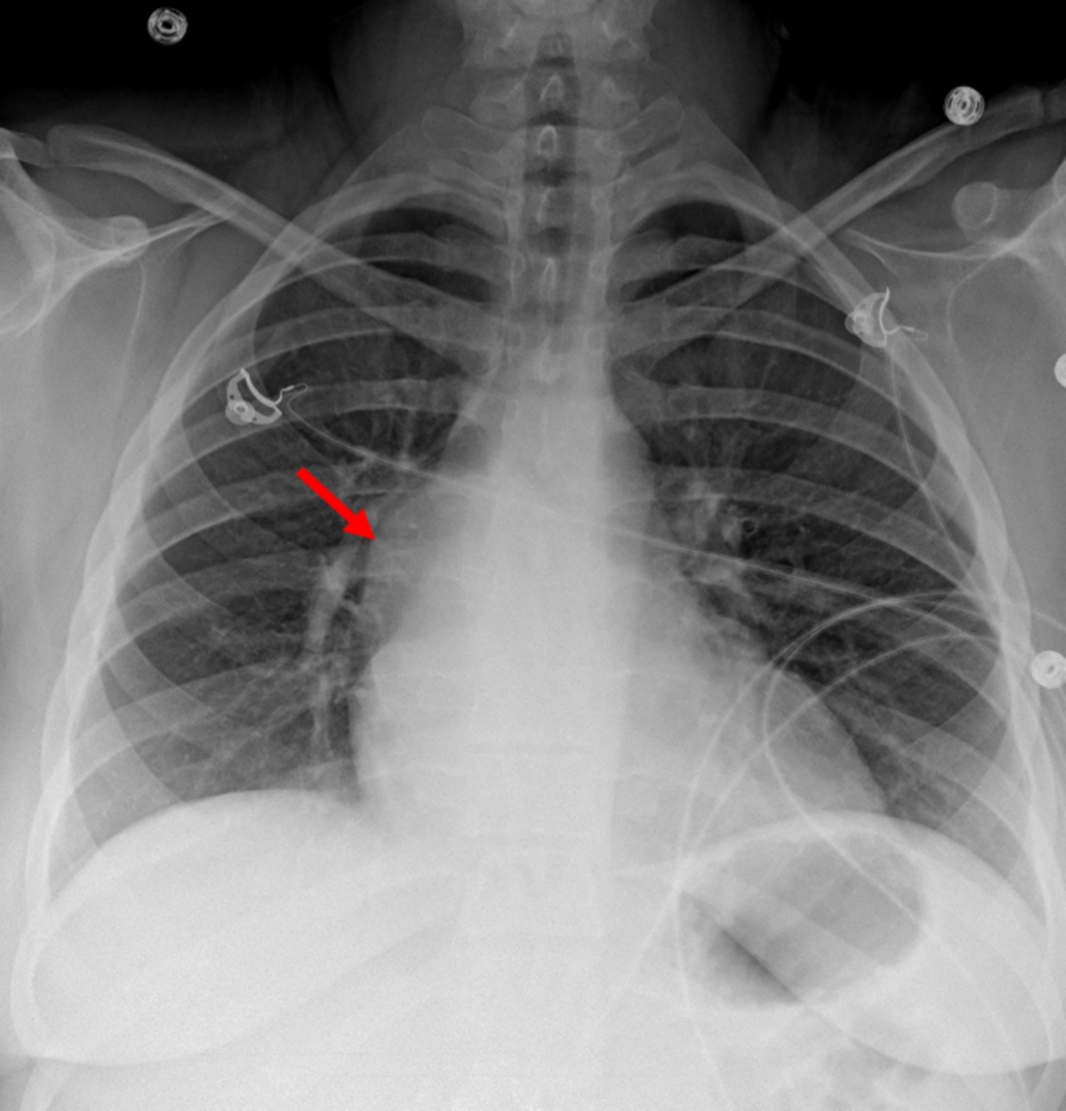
Chest X-ray (posteroanterior view) showing abnormal bulging of the right mediastinal contour (red arrow), suggestive of a mediastinal mass.
